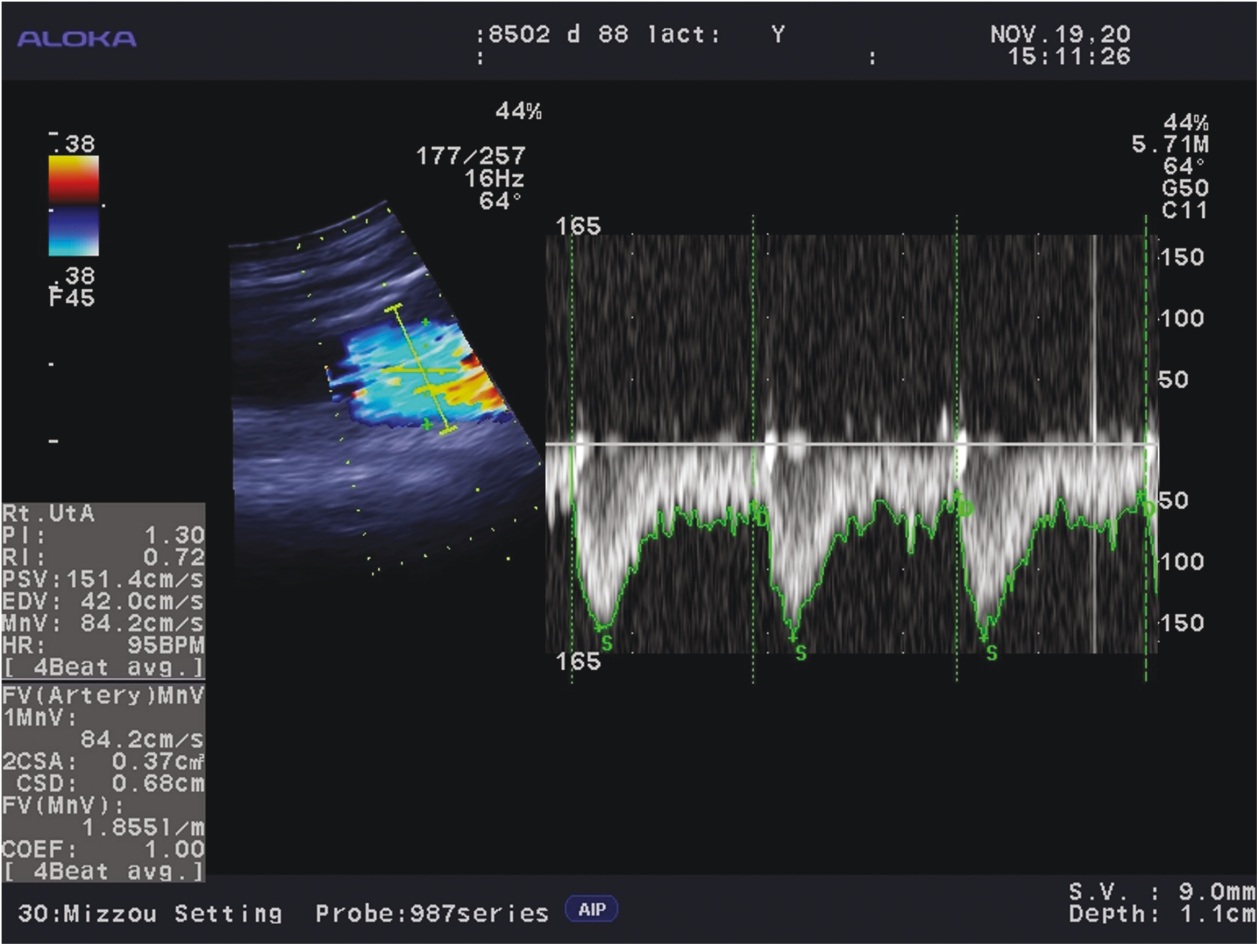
Color Doppler sonogram of the right pudendoepigastric arterial trunk of a primiparous beef female on day 88 of lactation. The image was obtained in B|D mode on an Aloka Prosound α6 machine after taking measurements chute-side. In B mode (left side), Doppler flow gain was set at 45, the angle of insonation (64°) was set parallel to the walls of the artery in a longitudinal view, and the caliper function was used to measure the cross-sectional diameter of the artery perpendicular to the angle of insonation. In D mode (right side), 4 representative and consistent cardiac waveforms were selected (only 3 are shown), from a similar set of frames as those from which the vessel was chosen. The machine calculated all variables, as displayed at the bottom left.
